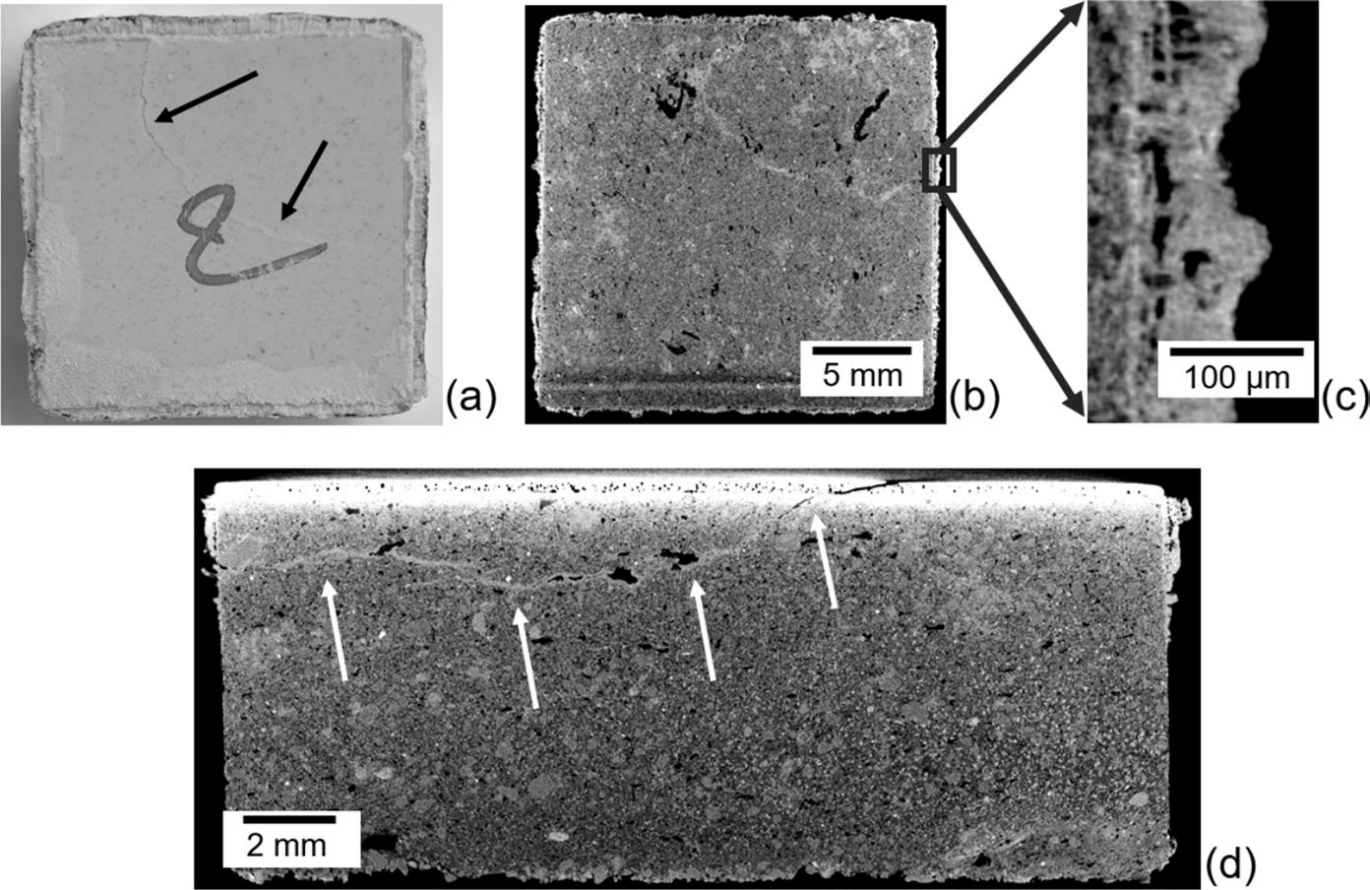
X-ray micro-computed tomography results at the end of the drying experiments on two-layered samples saturated with NaCl, adapted from [36]. a Initially intact tile sample damaged by NaCl precipitation (crack in the glaze highlighted by black arrows), b horizontal cross-section of the clay body, c salt crust at the border of the sample, d vertical cross-section of the sample showing damage induced by NaCl precipitation (crack in the body highlighted by white arrows)
